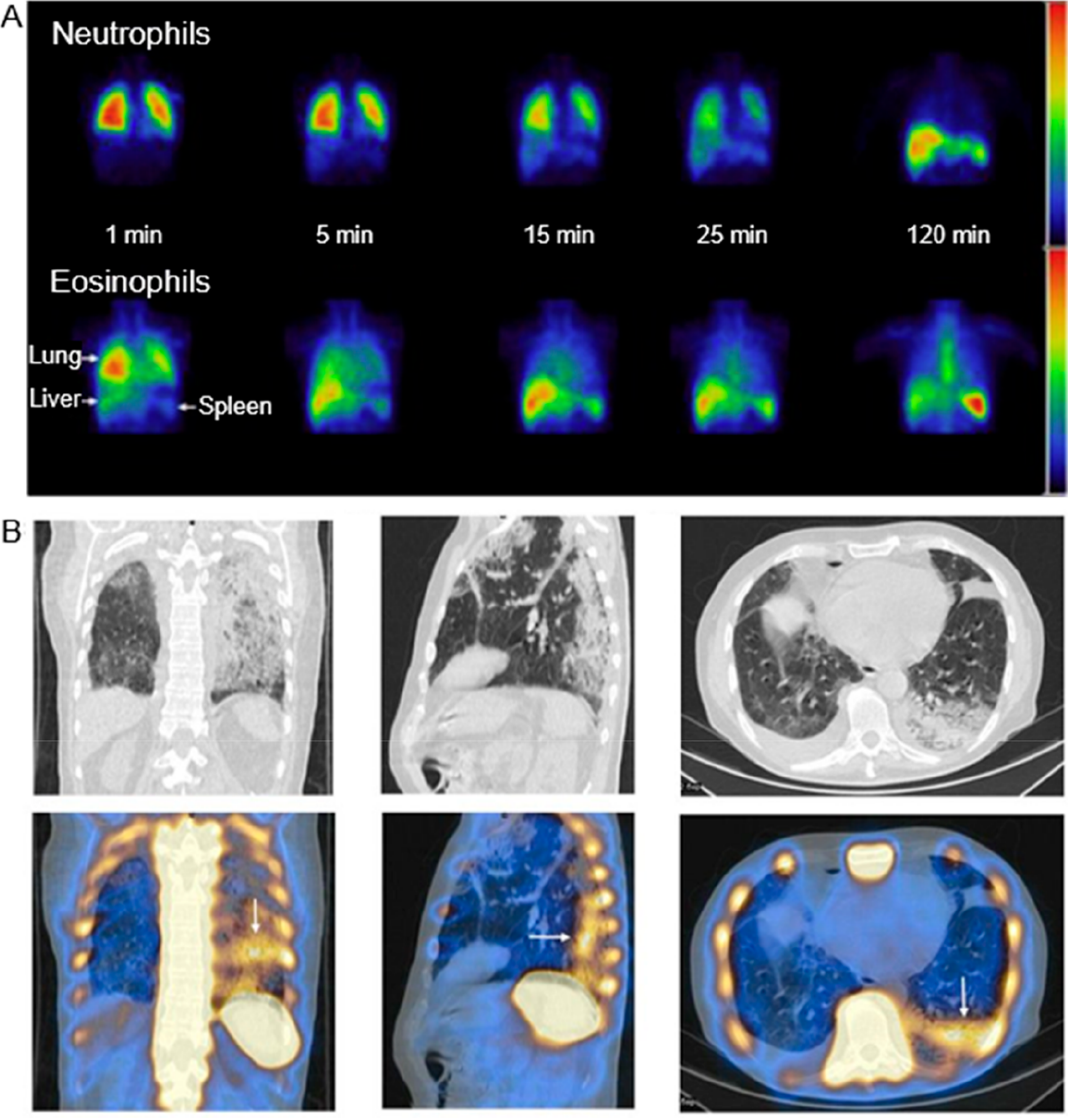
(A) Representative SPECT/CT images of affinity bead-purified neutrophils
